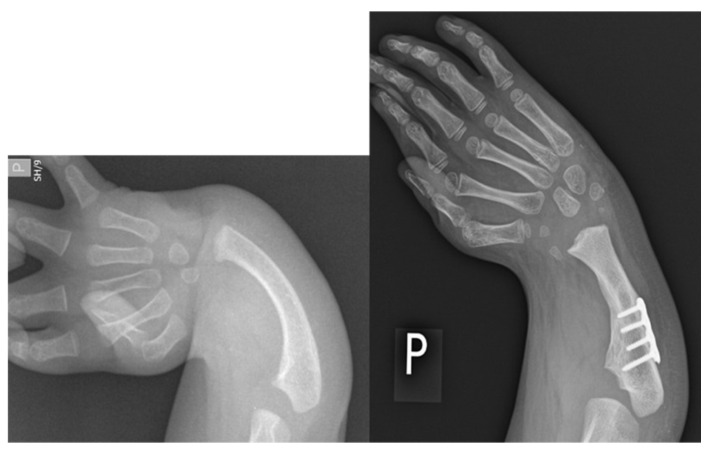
Case 6 preop radiographs (left); postop radiograph after ulnarization G3 (right). Note the hypertrophy of the head of the ulna after surgery.
